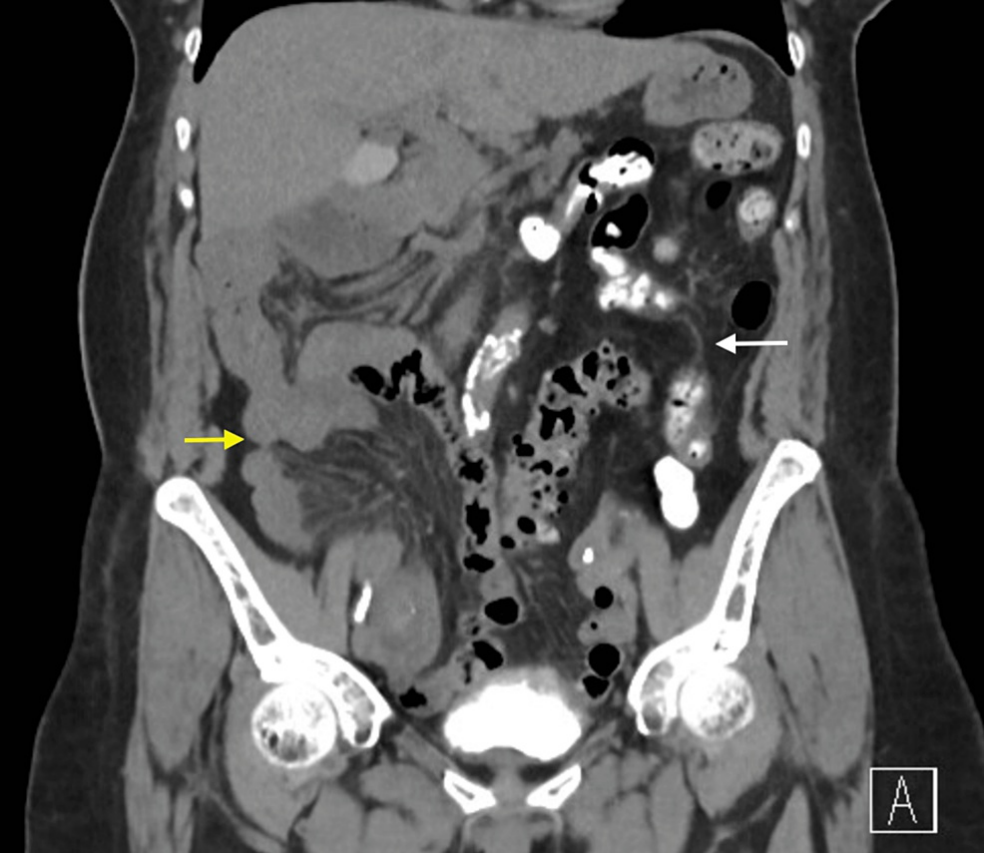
Coronal CT abdomen showing small intestine (yellow arrow) predominantly on the right side and the colon (white arrow) predominantly on the left side
